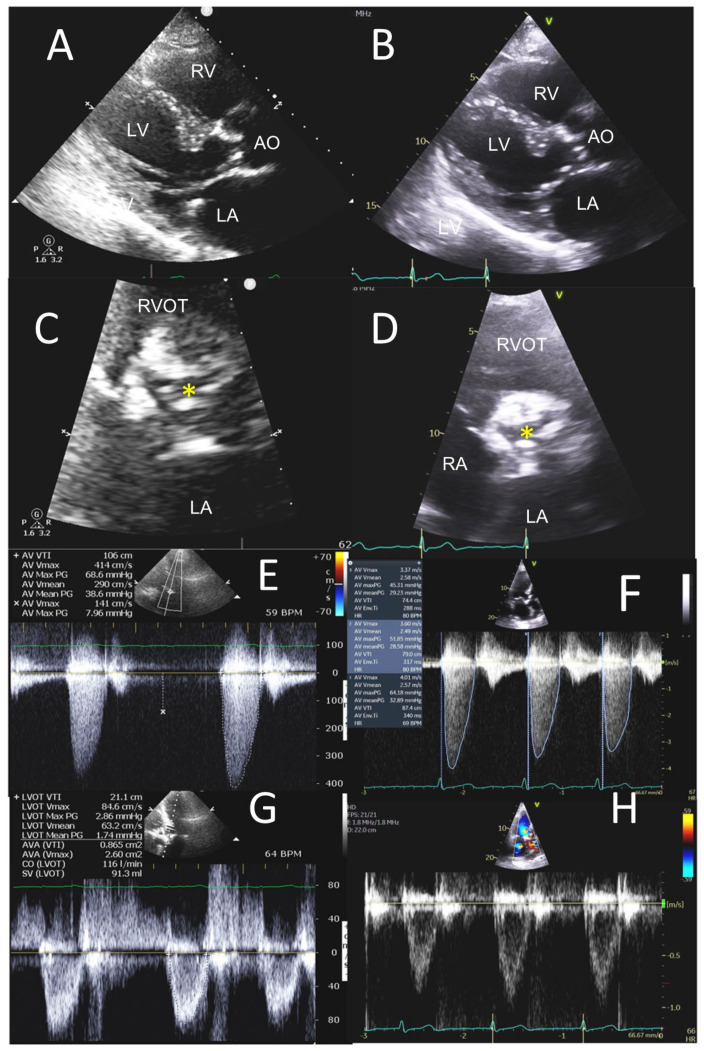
POCUS in stenotic aortic VHD. A 92-year-old male presented with exertional dyspnea and angina. POCUS images (left-side panels) are compared to the sTTE images (right-side panels). Parasternal long-axis views (A,B) show calcified aortic valve leaflets with restricted leaflet opening in systole parasternal short-axis views. (C,D) Heavily calcified aortic valve with marked restriction in leaflet opening and reduction in aortic valve orifice area (yellow asterisks (C,D)). CW Doppler in the apical five-chamber view (E) showed a mean gradient of 39 mmHg on POCUS, and mean gradient of 36 mmHg in the three-chamber view on sTTE (F). Aortic valve area was 0.87 cm^2^ on POCUS (G) and 0.88 cm^2^ on sTTE (H). Ao, aorta; LA, left atrium; LV, left ventricle; RA, right atrium; RV, right ventricle; RVOT, right ventricular outflow tract.
